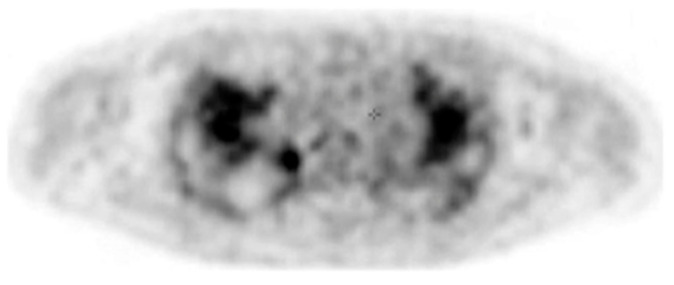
PET image at the thoracic level showing diffuse bilateral parenchymal FDG uptake. Reproduced from Mostard et al., 2011 [24]. Reprinted from Respiratory Medicine 105, 1917–1924 (2011) with permission from Elsevier.
